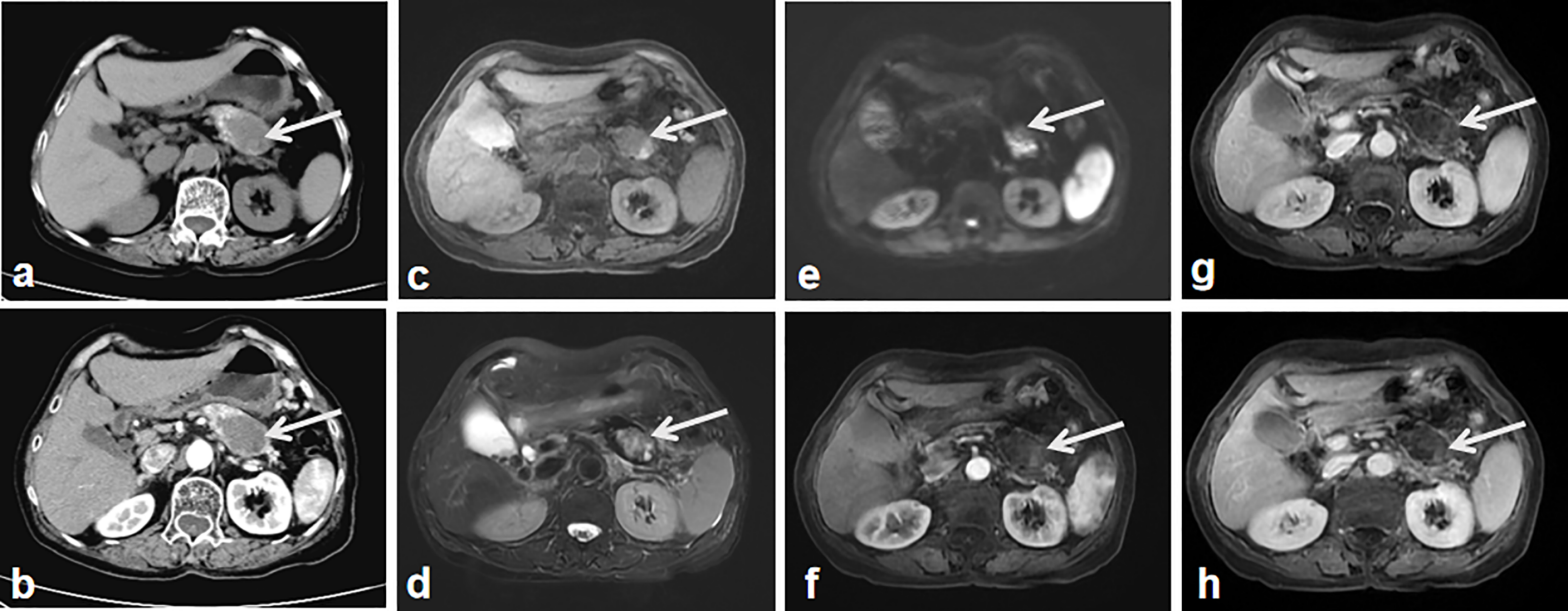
The second imaging. CT without contrast administration show the significantly enlarged tumor mass with heterogeneous components of central cystic low attenuation, peripheral solid isointensity and small patchy calcification (A, arrow). Contrast-enhanced CT shows the tumor mass with intermediate enhancement of the peripheral component and the non-enhanced cyst-like central area (B, arrow). MR T2 (C) and T2 weighted images (D) show heterogeneous hyper-signal intensity and hypo-signal intensity in the central area of the tumor (arrow). DWI shows the apparent diffusion restriction-induced heterogeneous hypersignal intensity (E, arrow). Contrast-enhanced MR weighted images of arterial (F), portal (G) and equilibrium phase (H) show the tumor mass with intermediate enhancement of the peripheral component, the non-enhanced cyst-like central area (arrows).
